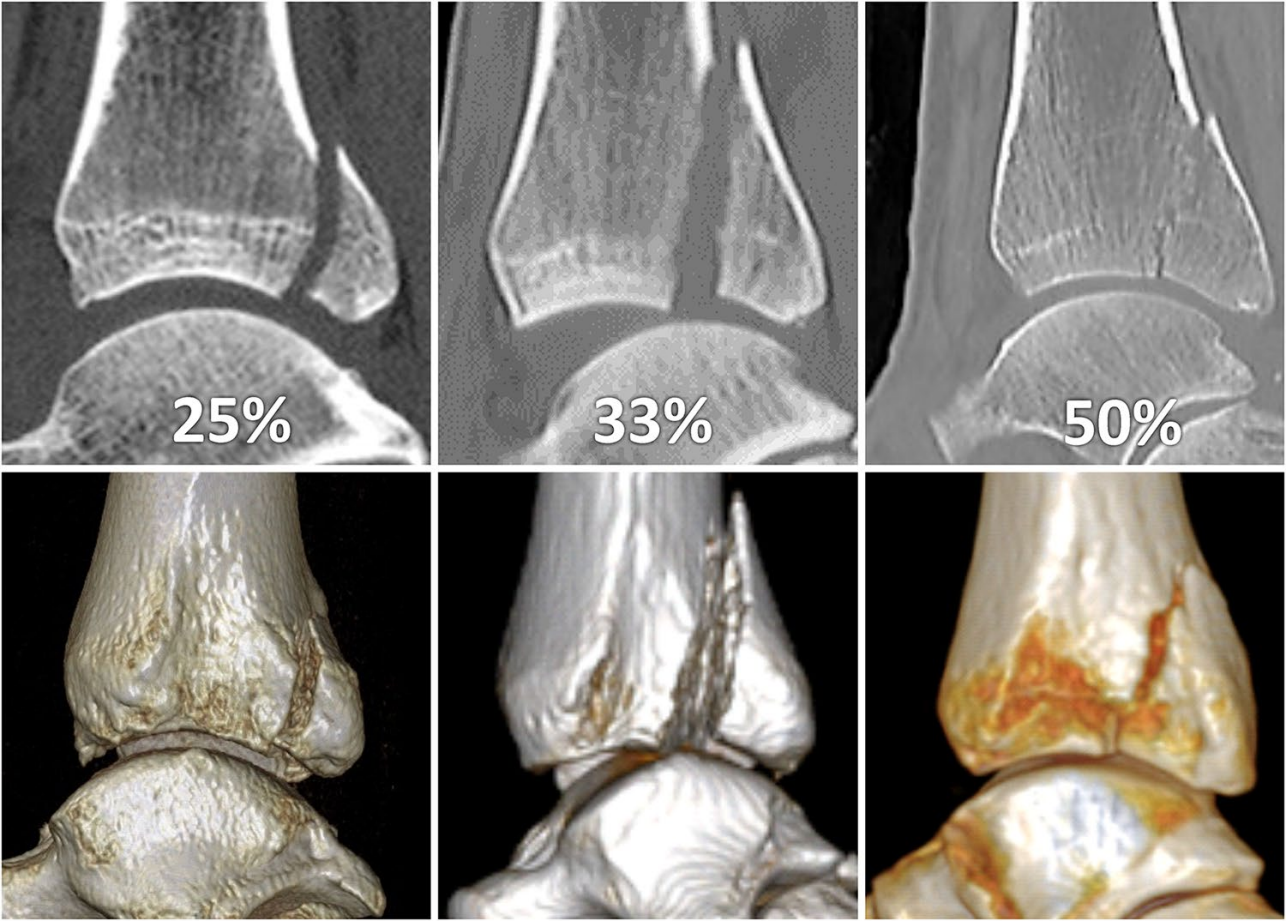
Increasing involvement of fibular notch by fracture of PM on 2D and 3D CT (lateral view with fibula subtraction) reconstructions
